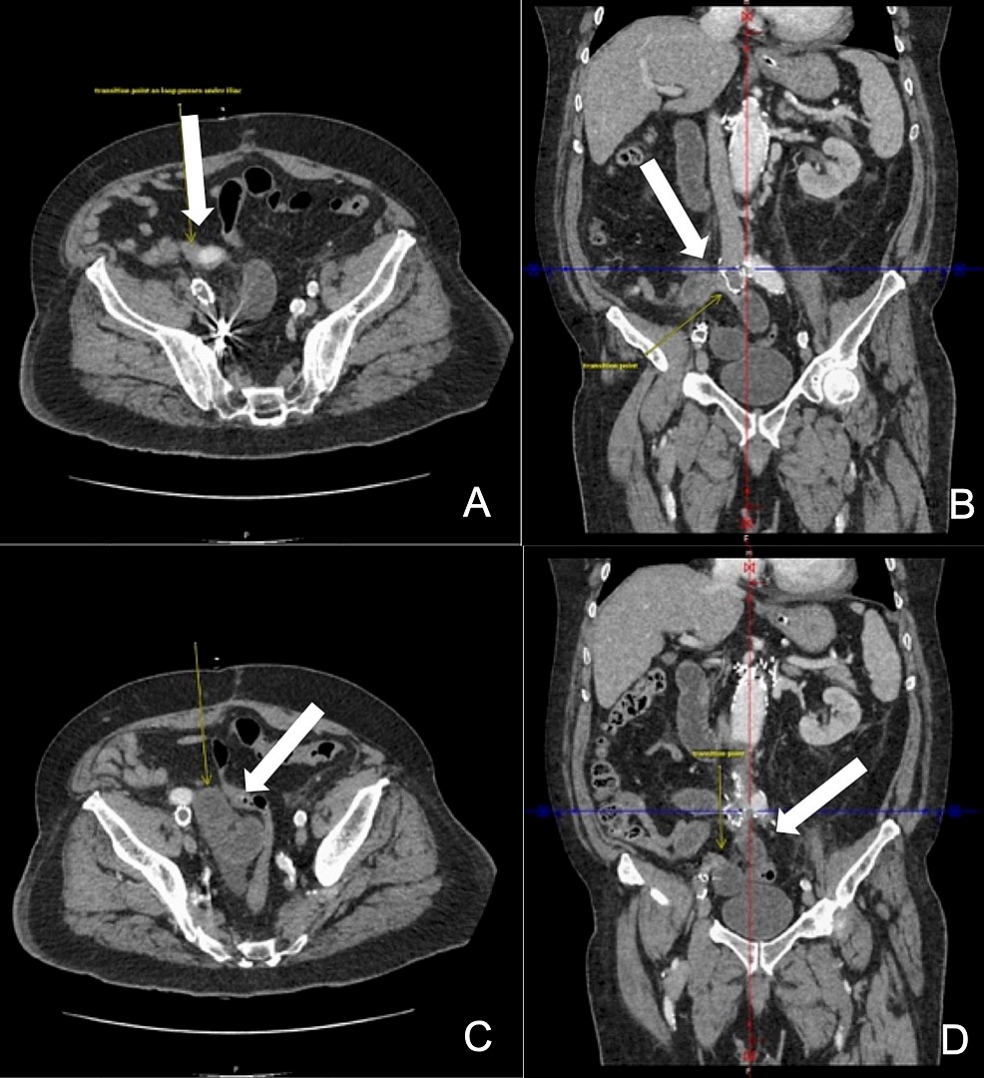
Computed tomography with intravenous contrast reveals a change in caliber as the bowel passes posterior to the right iliac graft limb (A,B). A dilated “C”- and “U”-shaped bowel loop can be visualized medial to the right iliac limb (C). Another transition point is seen in the more distal portion of the graft, consistent with closed-loop obstruction (D).
